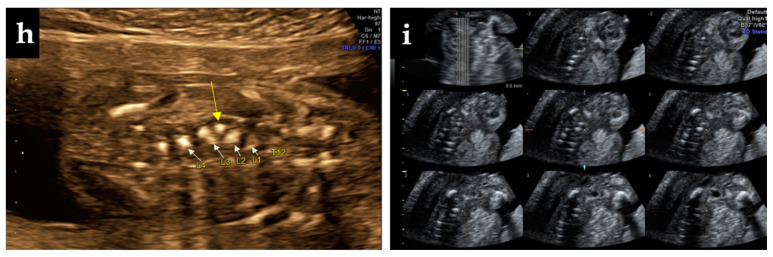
Ultrasound findings: (a) suspect angulation of the thoracolumbar spine at 12-weeks show the hemivertebrae (yellow arrow), (b) zoom-in above the hemivertebrae in 3D static reconstruction, (c) image of the whole spine at 12-weeks; (d) clear 2D imaging of the hemivertebrae; (e,f)—US scans of the fetal spine at 16 and 23 weeks, (g) tracing the correspondence of the vertebrae lateral ossification centers, (h) numbering and positioning of the vertebrae at 23 weeks on 2D ultrasound sepia mode, (i) Tomographic Ultrasound Imaging (TUI)—successive scans of the spine revealing the anomaly.
